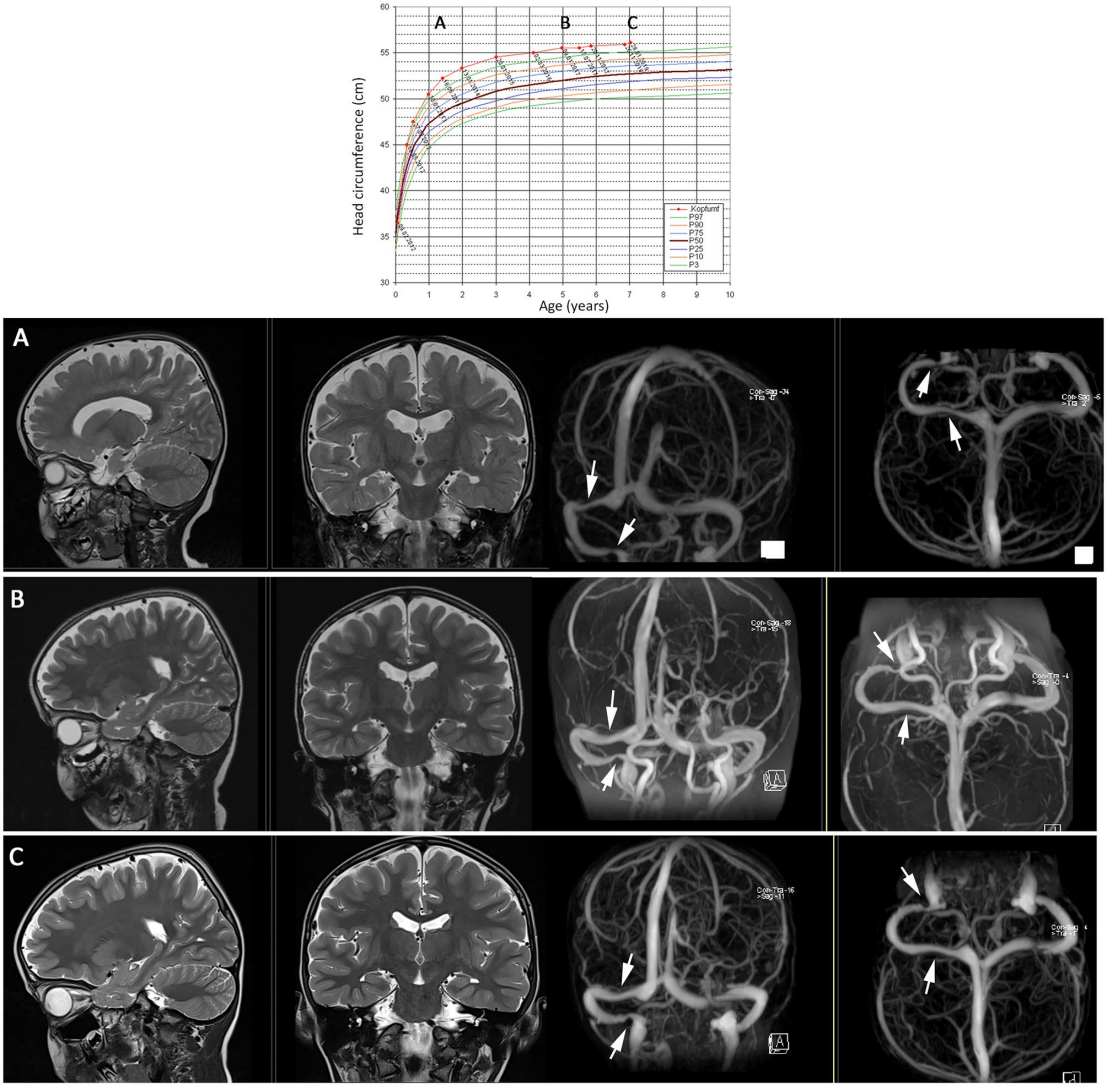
Typical course of head circumference (HC) and corresponding MRI controls during follow-up showing a slowly regressing CSAS in a persisting macrocephalic child with subarachnomegaly and venous sinus pathology. The top shows the head circumference curve over 7 years. The boy started with a HC at P50 at birth and developed a HC at P97 at 6 months of age. A At 1 y and 5 months, his HC was ~ 1 cm above P97 and the first MRI was performed. The ventricles are slightly larger without fulfilling the criteria for hydrocephalus. The frontal and parietal CSAS are enlarged, and the contrast-enhanced 3D venography shows a > 50% narrowing of the right transverse sinus, which was the direct drainage continuation of the sagittal sinus and a > 75% stenosis of the right sigmoid sinus immediately before the jugular foramen (white arrows). He maintained from that time point on his HC curve position about 1 cm above P97. B At age of 5 years, his CSAS have become smaller but are still wider than normal. The right transverse sinus stenosis has disappeared, and the pre-jugular foramen stenosis has remained unchanged. C The sinus situation is unchanged at age of 7 years; however, the l CSAS have decreases a little bit further
